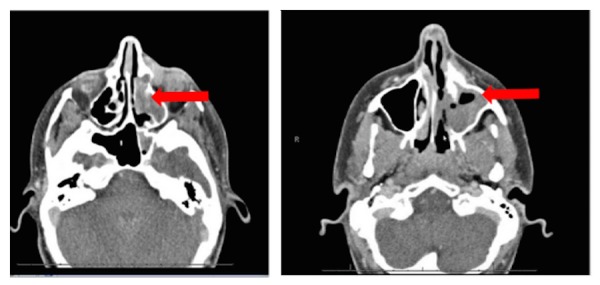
Presurgical CT Presurgical contrast-enhanced axial CT images of the head demonstrate an infiltrative mass in the left medial canthus region adjacent to the nasolacrimal duct, with resulting effacement of the left inferior meatus and partial effacement of the left middle meatus (see arrow). Also noted was adjacent hyperostosis along the anteromedial left maxillary sinus and additional involvement of the nasal cavity (see arrow).
